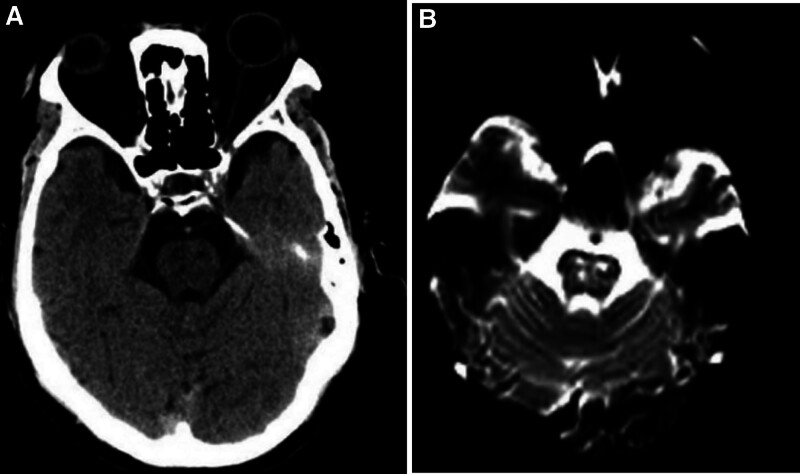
The brain computed tomography images show multiple small nodular low-density shadows in the pons (A) and the brain magnetic resonance image reveals punctate high-density shadows in the left pontine tegmentum (B).
